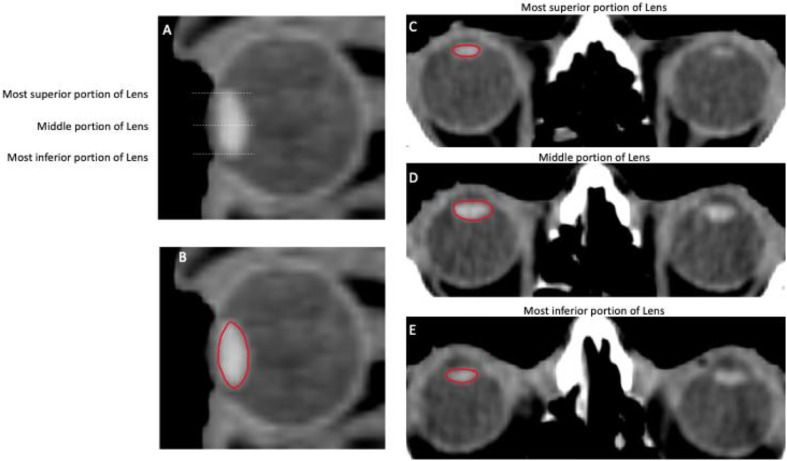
Computed tomography imaging of the globe for lens delineation. Midline sagittal view (A) and corresponding axial sections (C-E). The most superior portion of the lens in (A) aligns with axial section (C), the middle portion with (D), and the most inferior portion with (E). The red outline delineates the lens contour. Image (B) is identical to (A) but highlights the lens contour on the sagittal plane.
